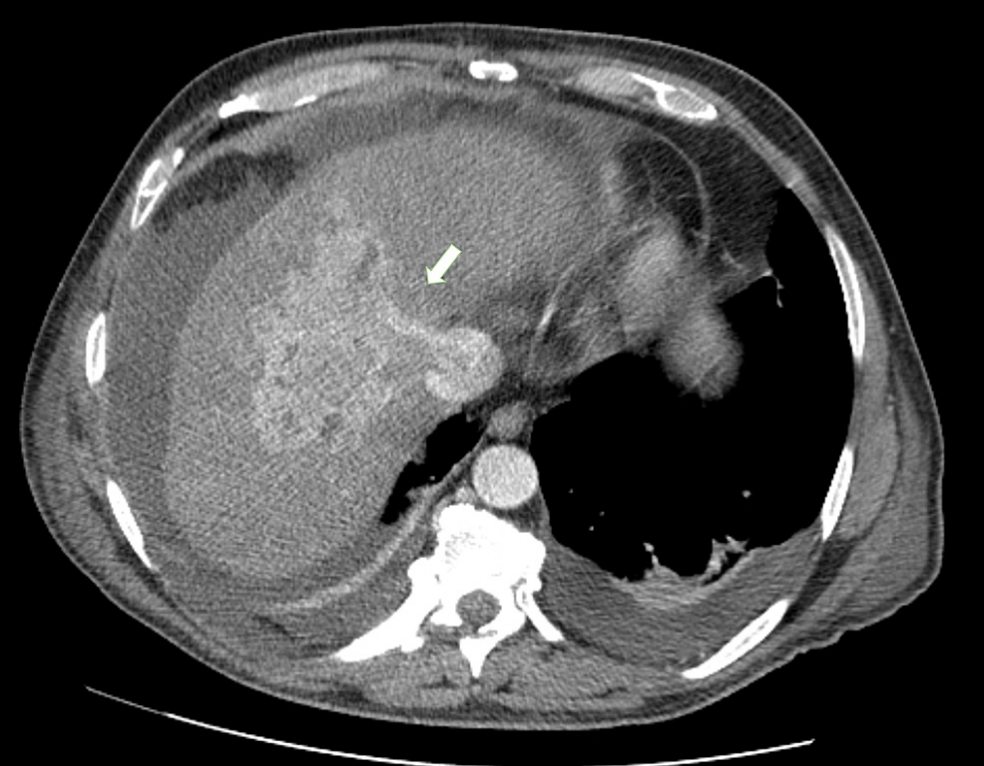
Computerized tomography (CT) with angiography with vena cava invasion Computerized tomography (CT) with angiography showing a liver mass compatible with hepatocellular carcinoma with vena cava invasion (arrow).
